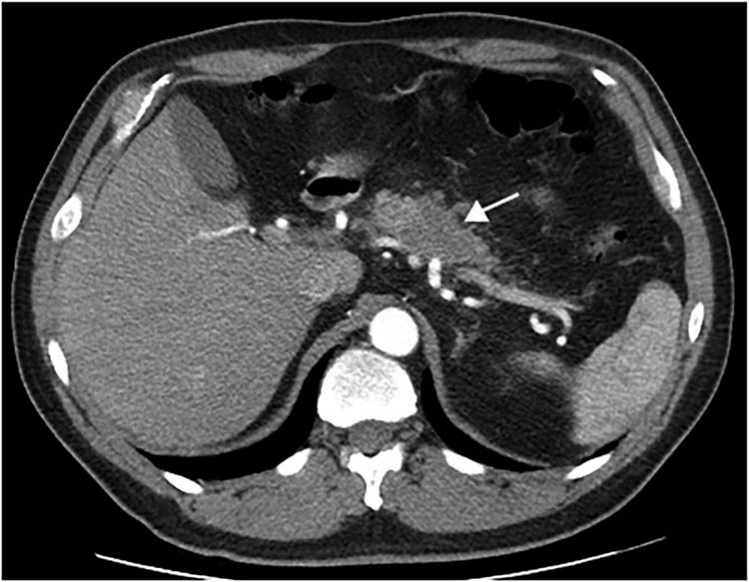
Preoperative CT-scan (arrow in the tumor).
